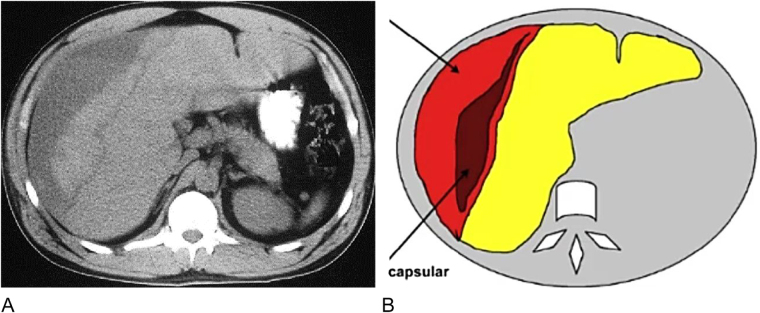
(A and B) Follow-up plain computed tomography (CT) of the abdomen and pelvis performed 1 month after presentation (9 October 2024), demonstrating a significant reduction in the size of the subcapsular hepatic hematoma and resolving hemoperitoneum.
